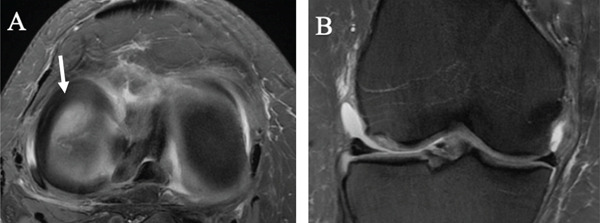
Preoperative magnetic resonance imaging of (A) axial and (B) coronal views of the right knee showing osteochondral impaction at the lateral femoral condyle (white arrow), with lateral meniscal tear at the posterior horn attachment and underlying bone marrow edema.
